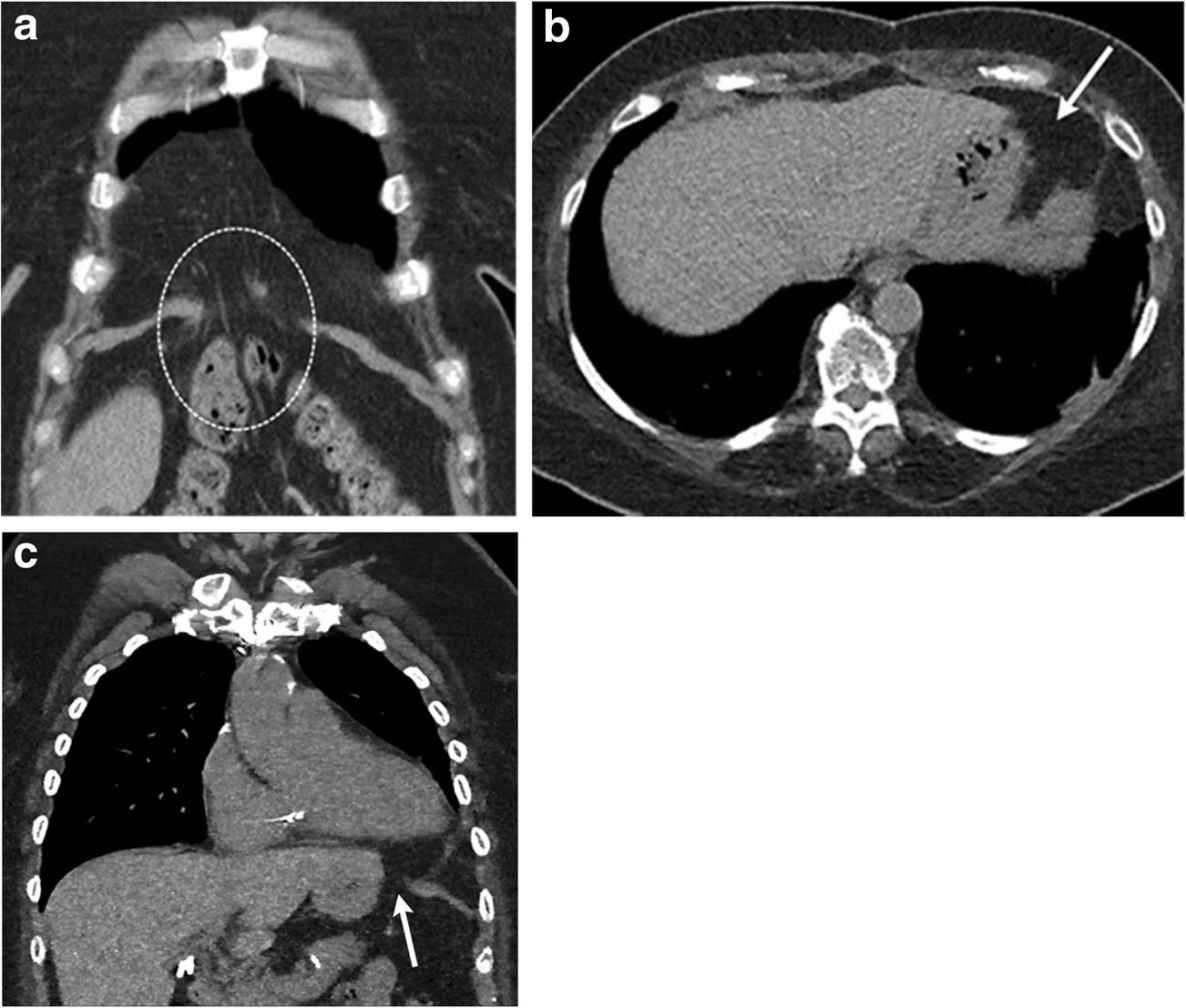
a A 46-year-old female being evaluated for right cardiophrenic angle opacity identified on a chest radiograph obtained during evaluation for pneumonia. Coronal CECT of the thorax identifies herniation of the omentum (dotted circle) through a clear defect in the right anterior-medial diaphragm (a) consistent with a Morgagni hernia. b, c A 49-year-old patient in the emergency department after trauma. Supine chest radiographs were suspicious for widening of the mediastinum. Axial (b) and coronal (c) CT clearly demonstrates a focal defect in the left anterior-medial diaphragm (white arrow) with herniation of peritoneal fat into the pericardium
